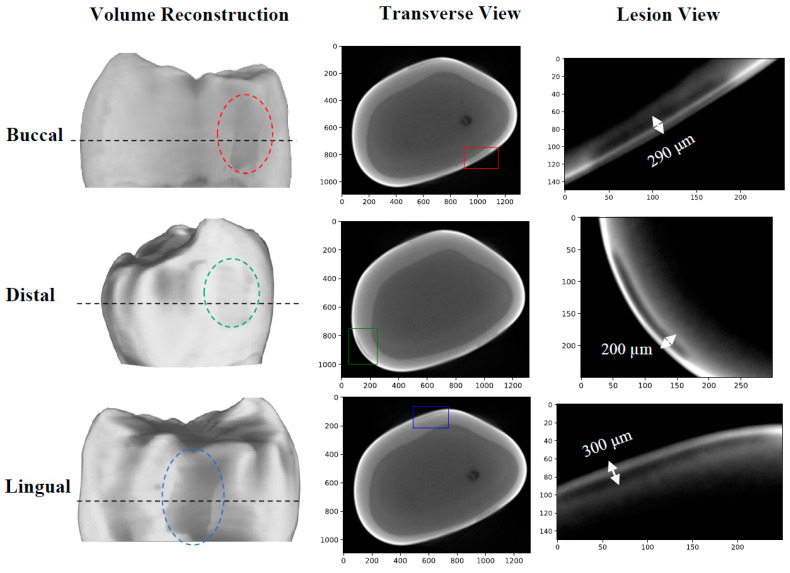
µ-CT images of the B8 sample after 8 days of cariogenic biofilm exposure: Three lesions are visible, one on each of the buccal, distal, and lingual surfaces. The dashed black line corresponds to the cross-sectional plane shown in the transverse views. The colored rectangles on the transverse views outline the position of the lesions. The “Lesion View” column shows an enlarged view of the lesions. To increase the visibility of the lesions, an adaptive histogram equalization was used [30] to improve the image contrast and visualize the edges of the lesion more definitively.
